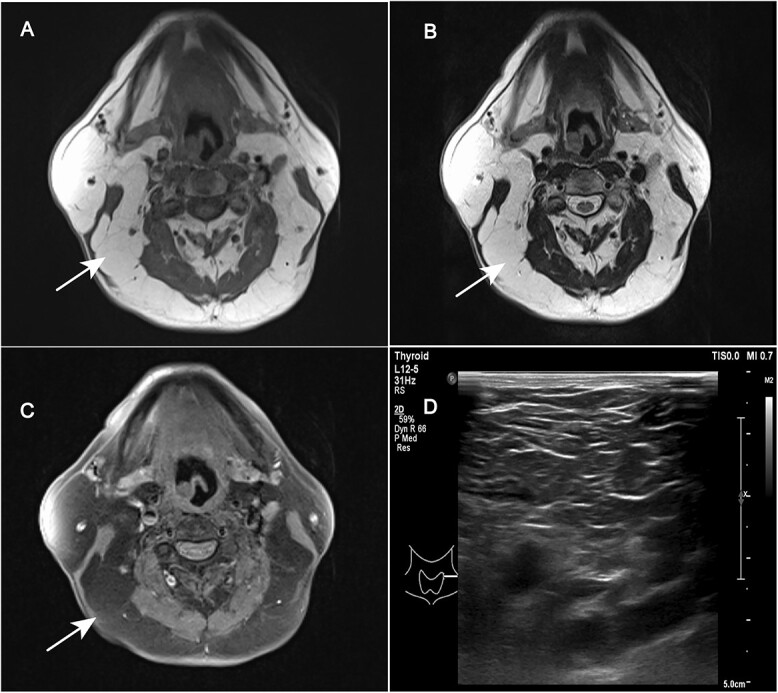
Magnetic resonance imaging of the head and neck: T1-weighted (A), T2-weighted (B) and fat suppressed imaging (C) showing non encapsulated soft tissue masses dispersed over the superficial and deep fascial spaces (Panel C, arrows). The color doppler ultrasound scan (D) of the masses showed irregular and diffuse thickening of subcutaneous fat tissue, without apparent capsules and borders.
